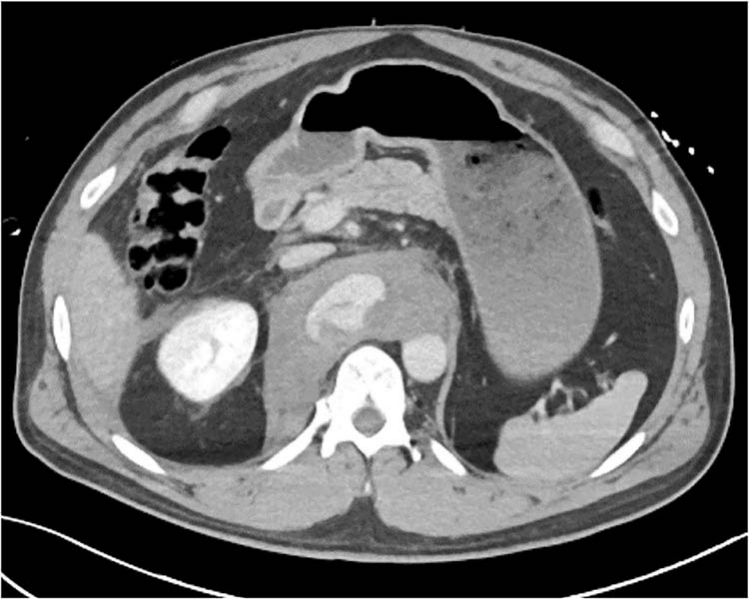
Axial CT slice demonstrating coeliac trunk avulsion with active bleeding into a large retroperitoneal haematoma.
